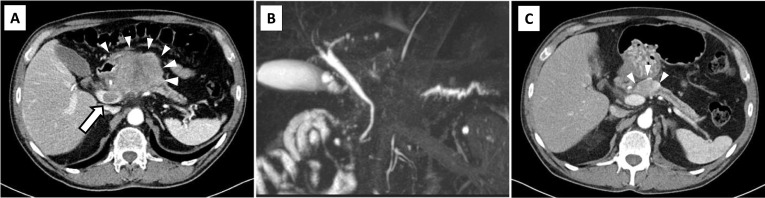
Computed tomography showed the pancreatic mass which was 7.5 cm in size at initial diagnosis (A, arrowhead). It revealed portal vein embolism (A, arrow), which was suggestive of tumor emboli at the main trunk and stenosis of the main pancreatic ducts with distal dilatation of the pancreatic duct (A). Magnetic resonance cholangiopancreatography exhibited the main pancreatic duct (MPD) stenosis at the pancreatic head with distal dilatation of MPD (B). After ten cycles of chemotherapy with FOLFIRINOX, the tumor shrunk to 3 cm (C, arrowhead), and the thrombi in the main portal vein could not be identified (C)
